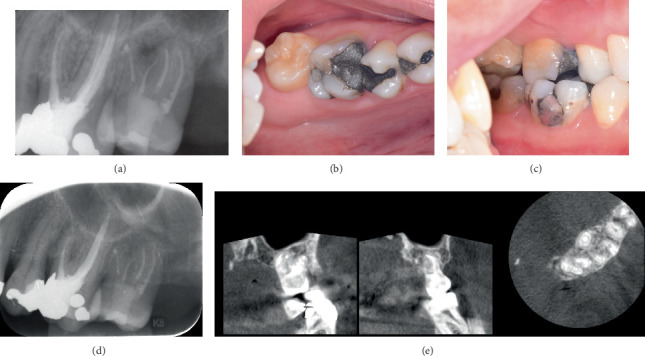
The postoperative care and final follow-up. (a) A periapical radiograph was taken on the day of the root canal treatment. (b, c) The final follow-up, conducted 18 months after the surgery, confirmed that the tooth remained functional and clinically asymptomatic, without the need for additional restoration. (d) Radiographic examination revealed no signs of complications. (e) Additionally, a CBCT scan was conducted to properly evaluate the healing of both the resected root and the previously damaged root surface.
